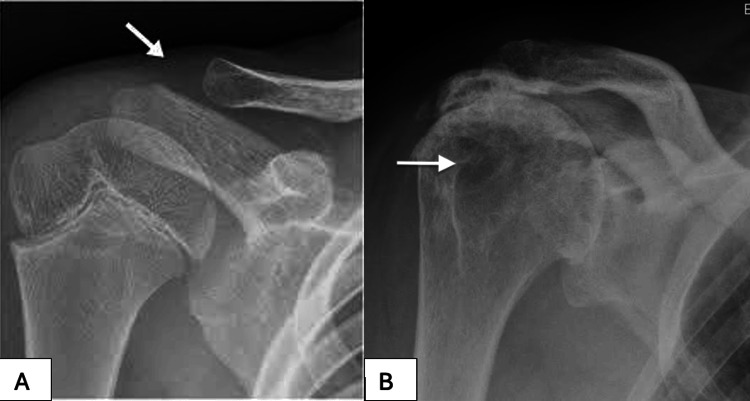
X-ray anteroposterior view of the right shoulder. (A) Erosion of the acromioclavicular joint (white arrow). (B) Evidence of a round radiolucent area seen in the humeral head, suggestive of cyst (white arrow). Surrounding soft tissue appears normal.
